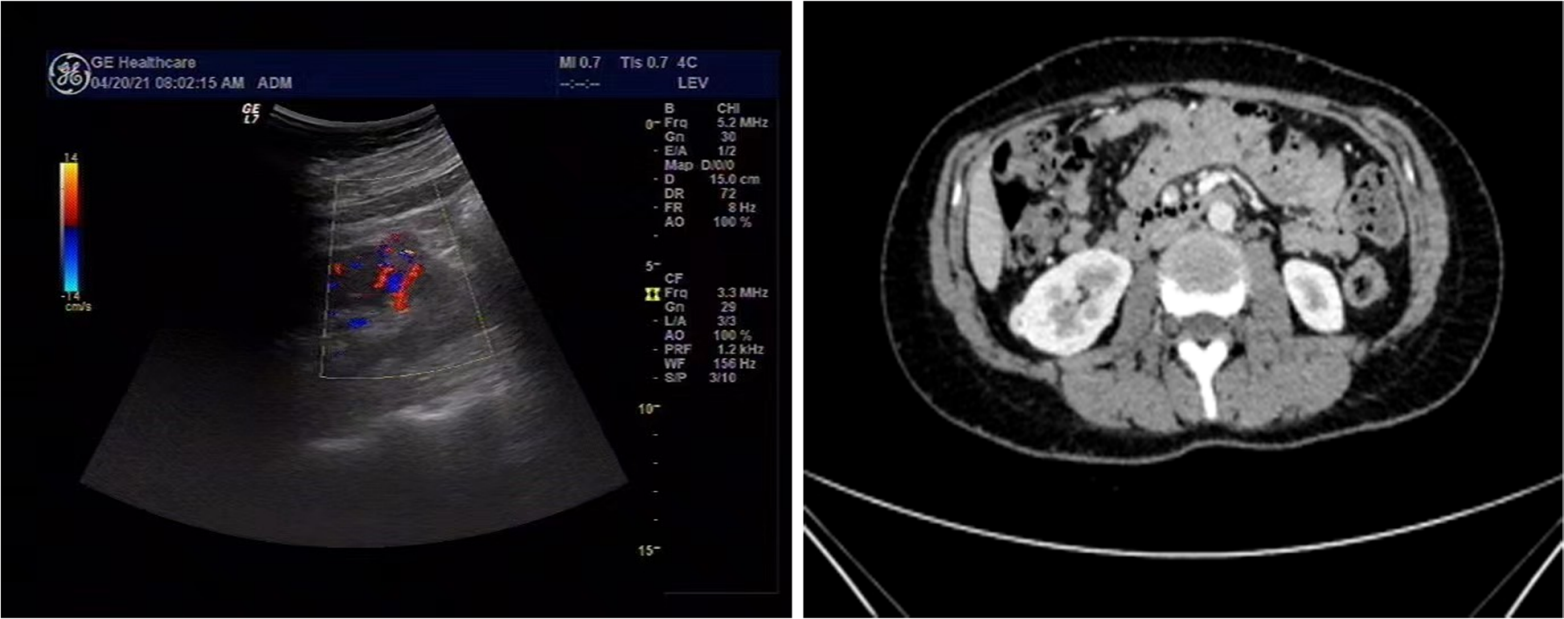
Imaging characteristics of the tumour. A Ultrasound image of this case. Ultrasound showed an isoechoic area at the lower pole of the right kidney, with a size of 1.6 × 1.3 cm, a clear boundary, and an uneven echo; it was slightly convex locally, and the blood flow signal could be seen. B Enhanced CT of the lower abdomen displayed a solid space occupying the right kidney
